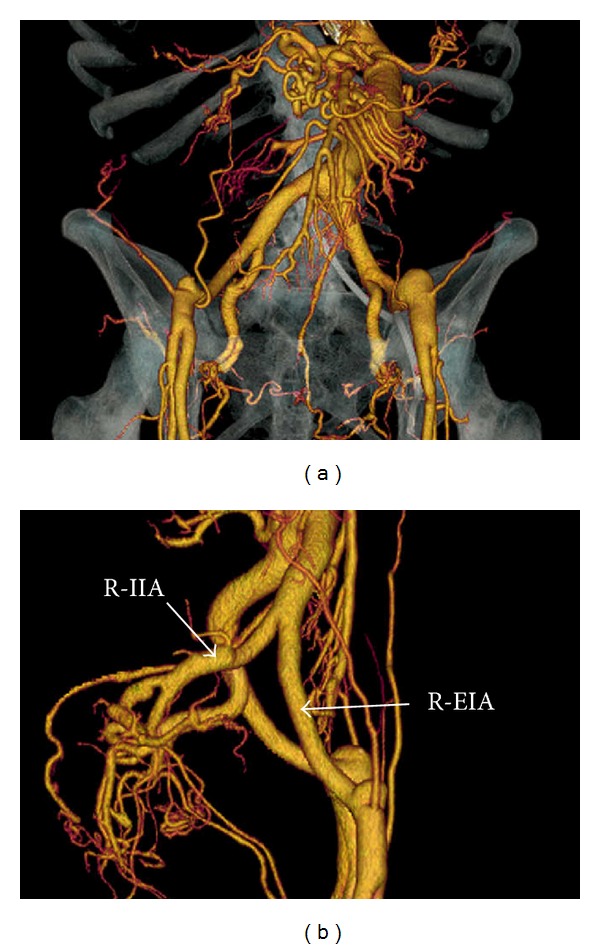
Three-dimensional computed tomography scan demonstrating no dissection or aneurysm in the iliac and external iliac arteries. (a) Anterior to posterior imaging. (b) Right-to left-sided imaging. R-IIA: right internal iliac artery; R-EIA: right external iliac artery.
